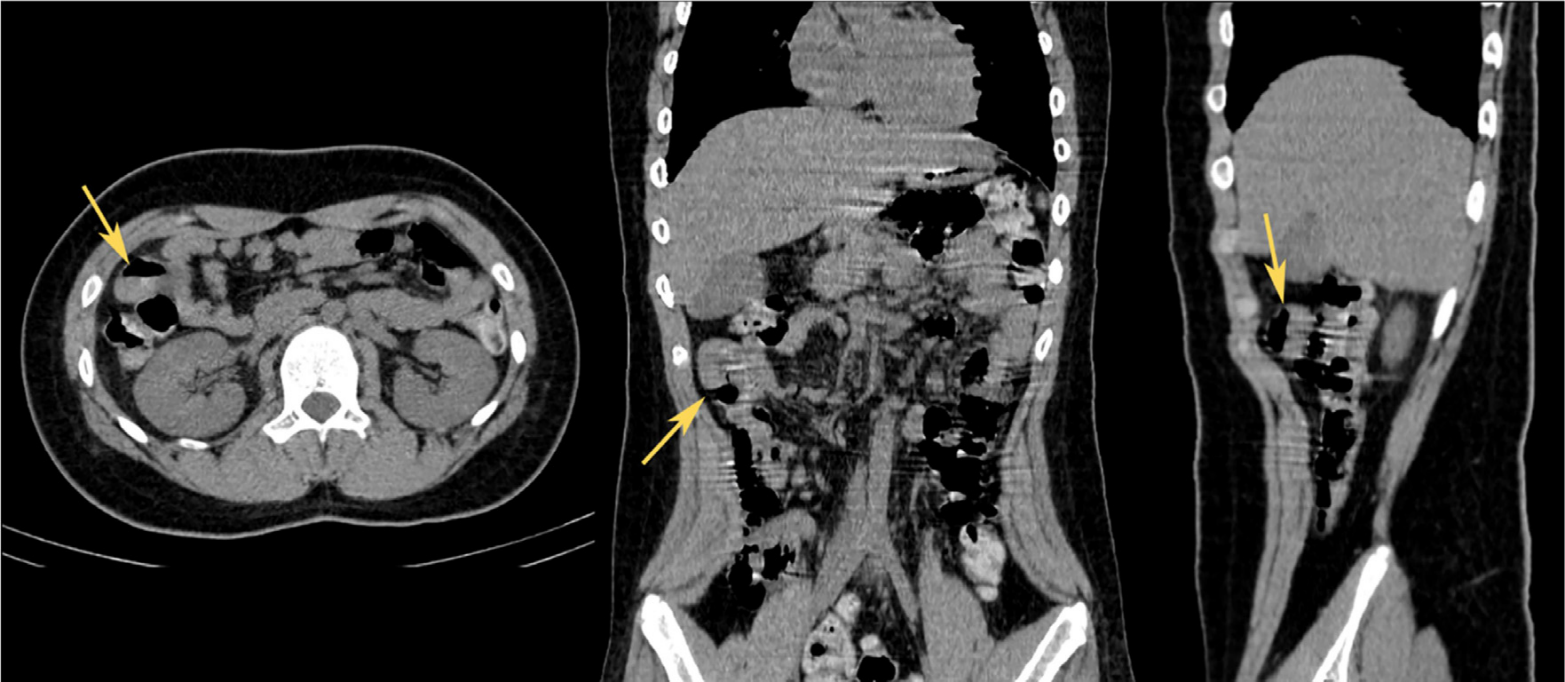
A non-contrast CT scan with axial, coronal, and sagittal images only showed a thickened intestinal mucosa (marked by arrows).
